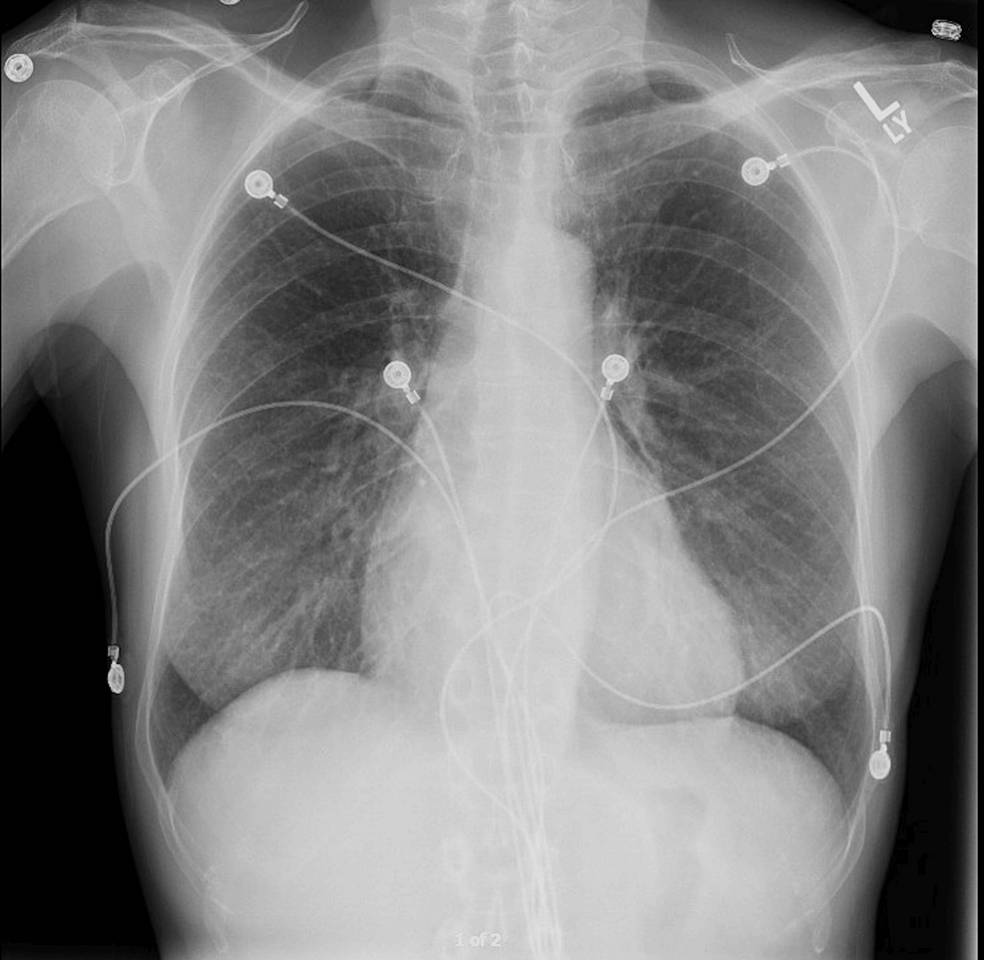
Chest X-ray on admission showing mild cardiomegaly. Image obtained from the patient’s chart.
